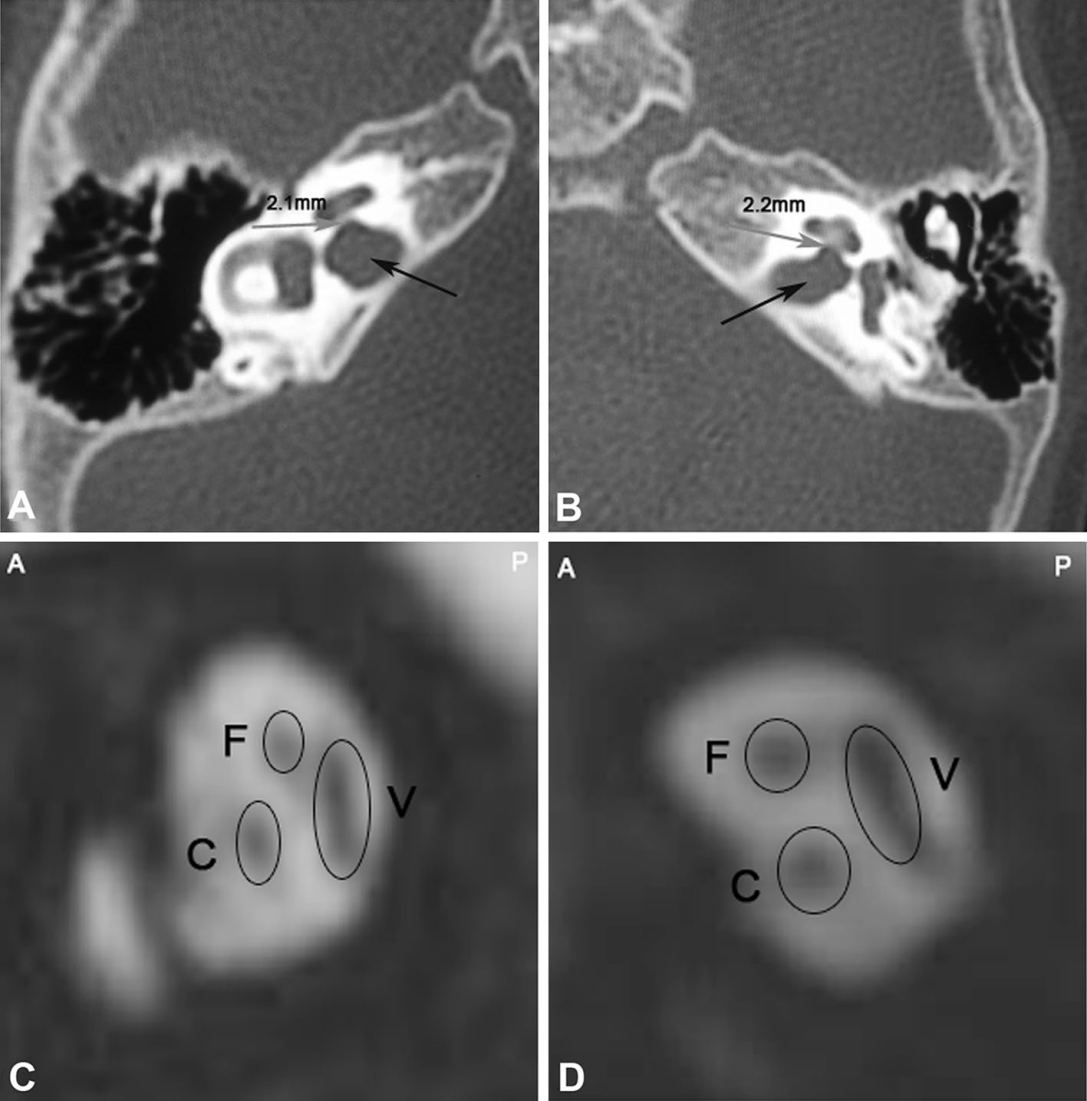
Enlargement of the IAM, with normal CN. Female, 2 years old. Bilateral severe hearing loss. a, b Axial CT shows an enlarged IAM. The CNC on the right side is 2.1 mm (a); 2.2 mm on the left side (b). c, d Oblique sagittal reconstruction of an MRI image shows normal development of the facial nerve in the IAM, the vestibular nerve, and the CN
